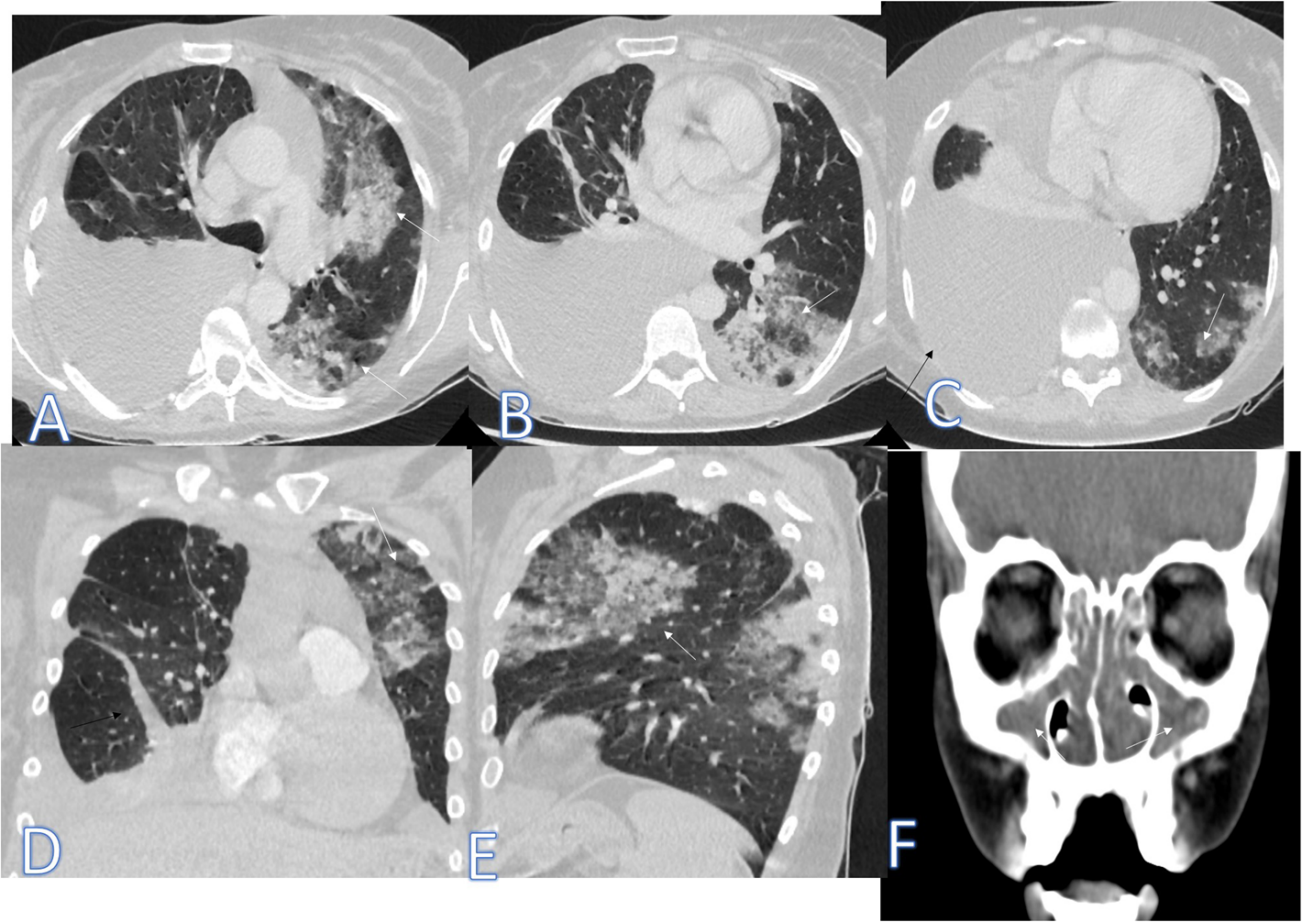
A 39-year-old asthmatic female patient presented with shortness of breath with cough and dyspnea. CT showed multiple consolidative patches with GGO and crazy paving appearance (white arrows) and moderate right pleural effusion (black arrow). CT paranasal sinus (f) showed bilateral total opacifications of the sinus (arrow). The diagnosis was Churg-Strauss syndrome
